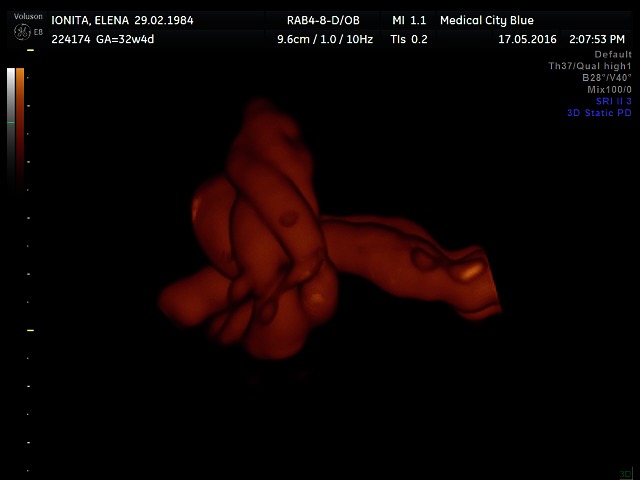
True umbilical cord knot on 3D power Doppler
